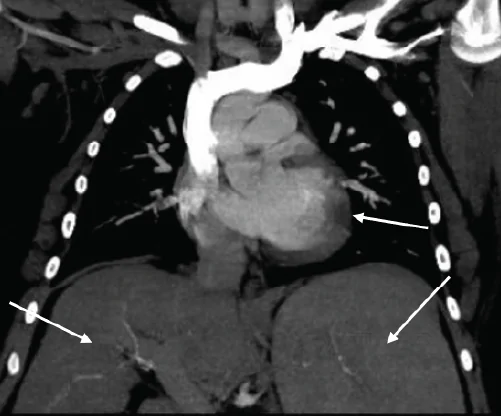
Initial computed tomography angiogram of the chest demonstrating a cardiac silhouette at the upper limits of normal in size (upper arrow) and partial visualization of hepatosplenomegaly (lower left and right arrows).
